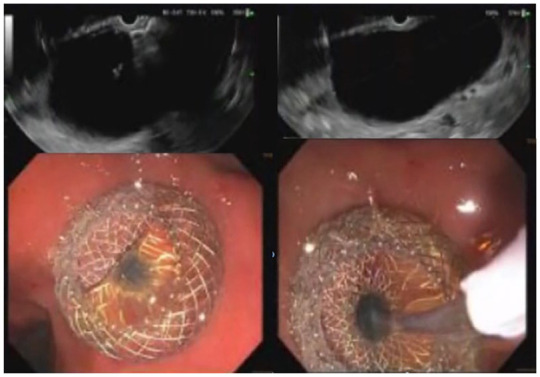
Transmural EUS-guided LAMS placement in the paragastric loculated malignant ascites. EUS, endoscopic ultrasound.
